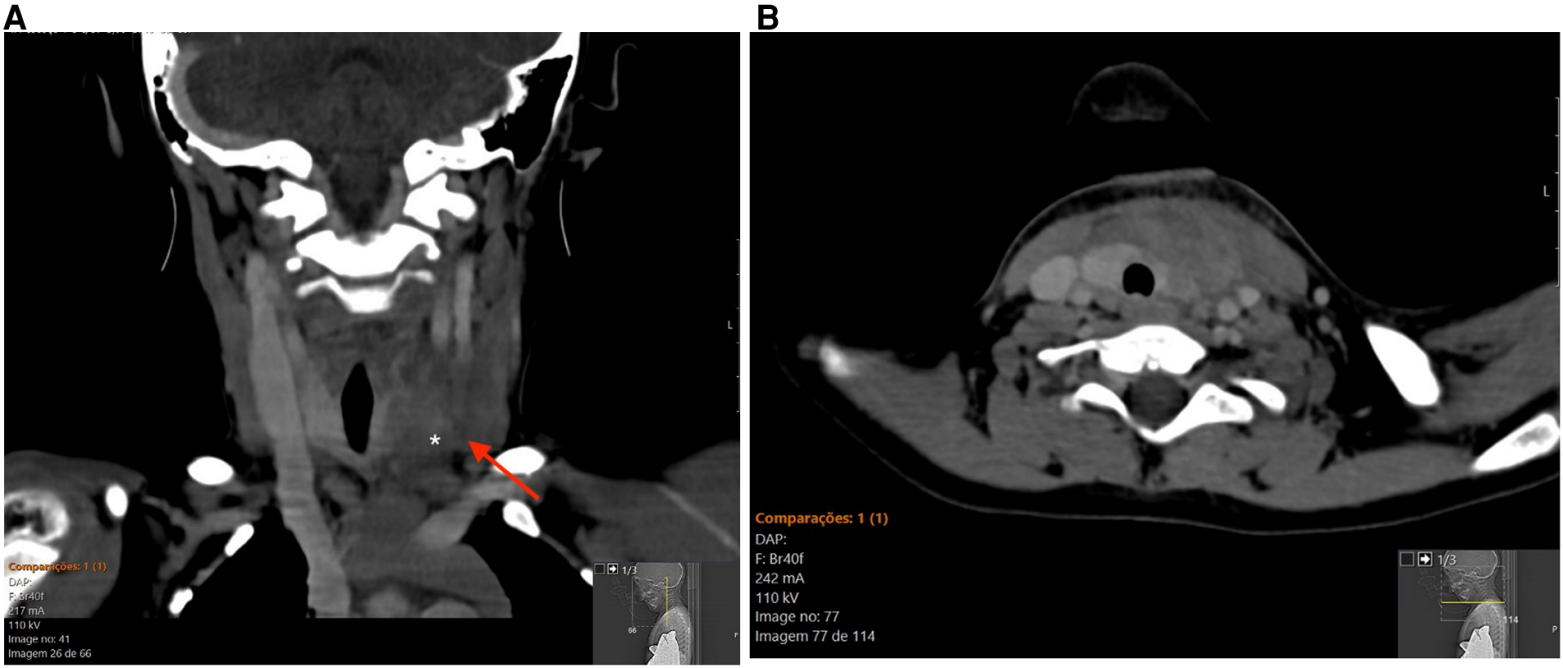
Contrast-enhanced CT of the neck. (A) coronal reformat displaying a ill-defined inflammatory mass (arrow) infiltrating the left lobe of the thyroid gland. (B) corresponding axial CT image.
